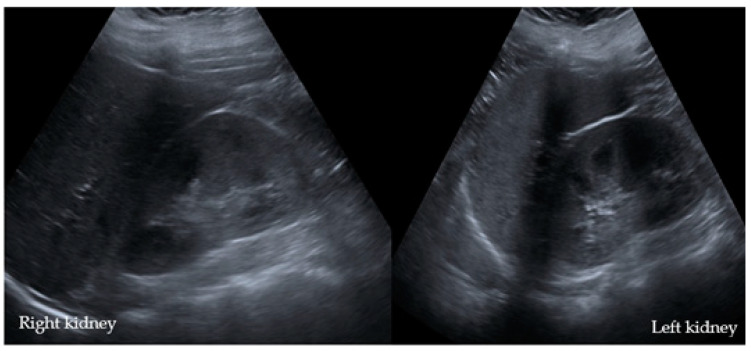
An ultrasonography of the kidneys of Case II°. Both kidneys presented mild echogenic accentuation of the parenchyma with consecutive increased corticomedullary differentiation.
